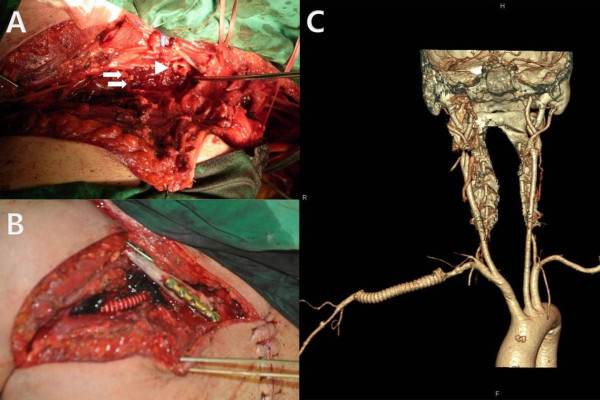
Open surgical repair. A: Multiple venous openings (white arrows) were observed in the pseudoaneurysmal sac. B: The subclavian artery pseudoaneurysmal sac was removed, and graft interposition was performed on the subclavian and axillary arteries with an 8-mm Ringed Gore-Tex ® Vascular Graft. C: Follow-up 3D CT angiogram shows good distal blood flow to the axillary artery through the subclavian artery graft.
